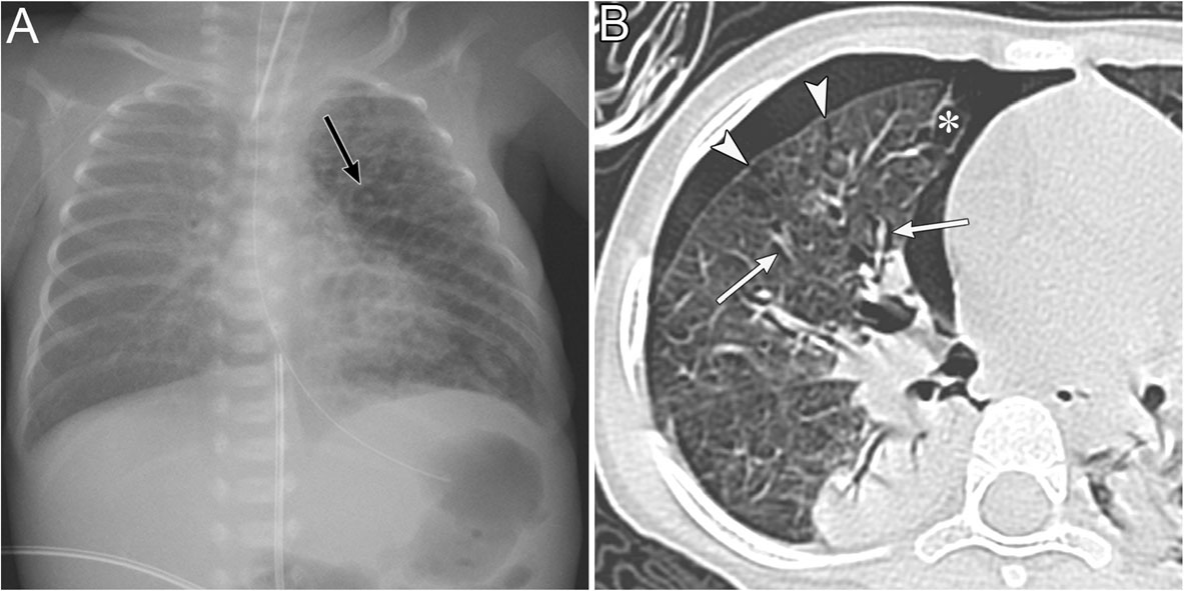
Pulmonary interstitial emphysema. a A supine radiograph of a 6-day-old girl with an extremely low birth weight shows generalized irregular radiolucent mottling and perivascular air (black arrow) in the left lung. b An axial chest CT image of a different patient (a 20-month-old girl with pneumonia) shows a subpleural cyst (asterisk), air within the interlobular septa (arrowheads), perivascular and peribronchial emphysema (white arrows), and pneumothorax
